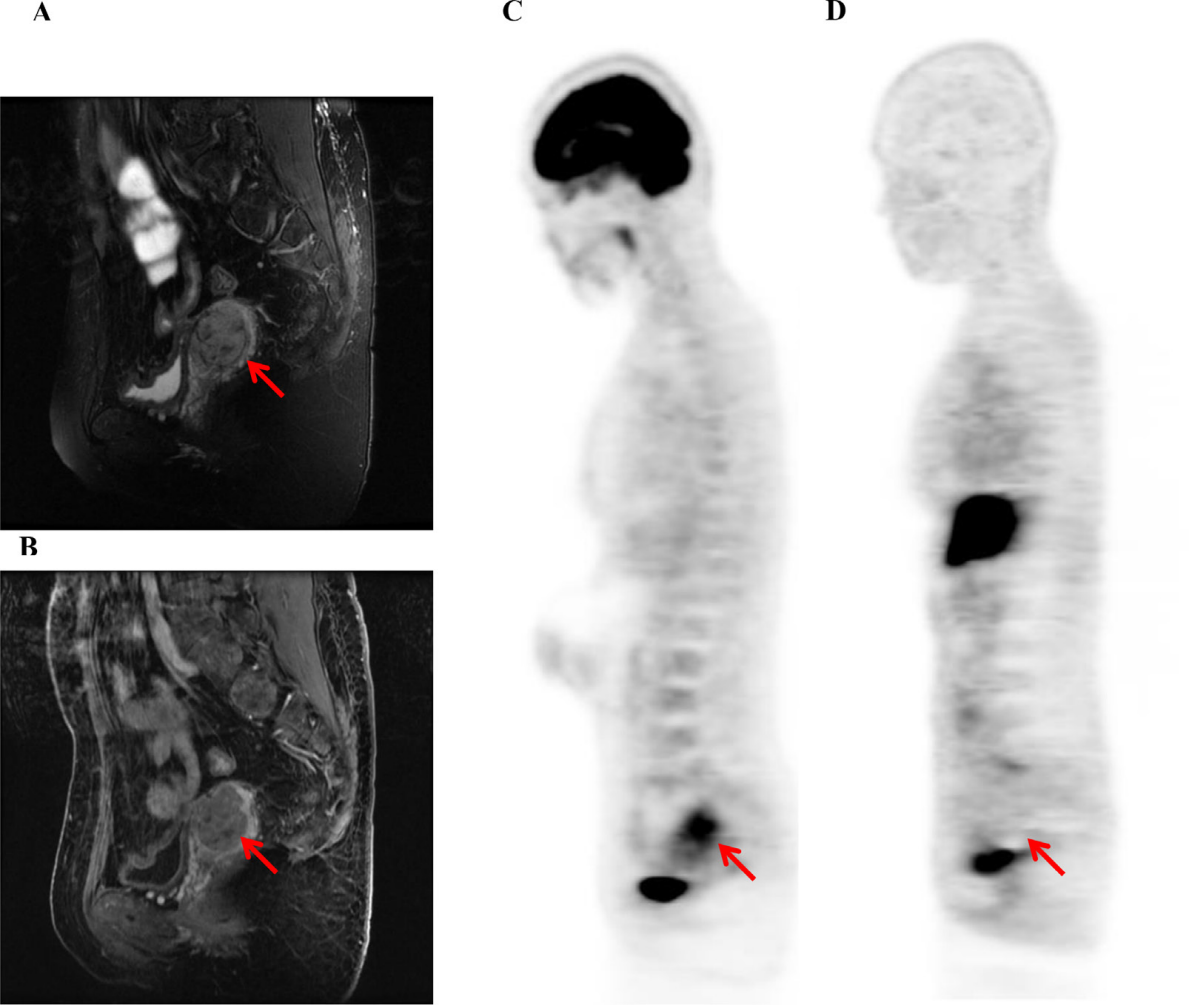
A representative case of uterine leiomyosarcoma (LMS, 41-y-old patient, FIGO stage I) T2-weighted (A) and contrast-enhanced T1-weighted (B) MR images and ^18^F-FDG (C) and ^18^F-FES (D) PET images are shown. The ^18^F-FDG SUV, ^18^F-FES SUV, and ^18^F-FDG/^18^F-FES SUV ratio were 7.0, 1.1, and 6.4, respectively. This patient also underwent TAH + BSO; however, she had local recurrence and lung metastases 2 months later and died 8 months later.
